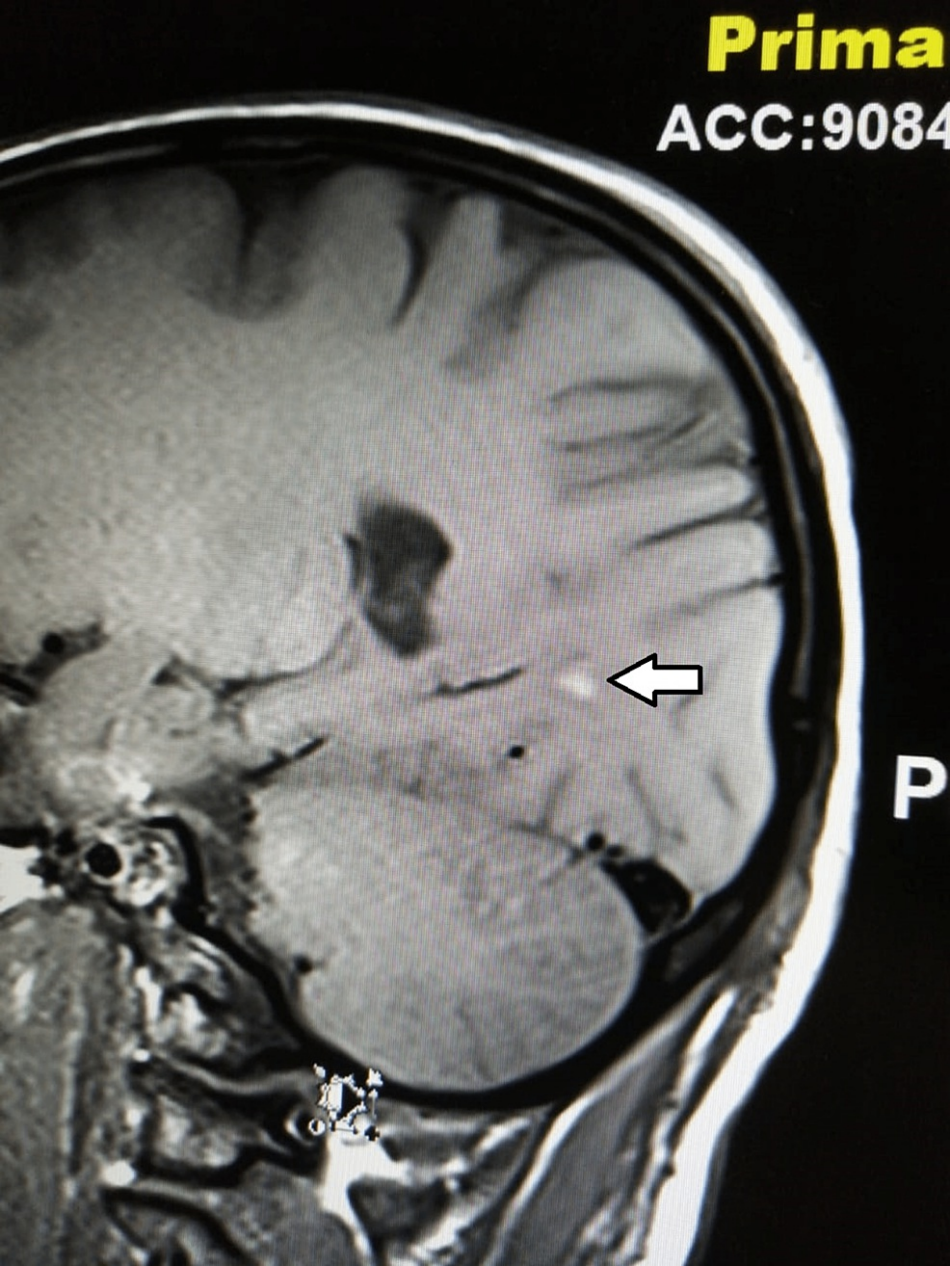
T1-weighted MRI image The image shows a hyperintense focus in the white matter of the right occipital lobe, representing a tiny hemorrhage (white arrow).
